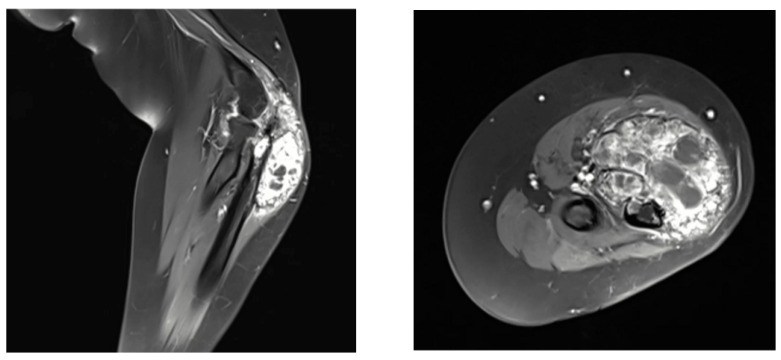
Magnetic resonance imaging (MRI) of the right forearm showing the giant cell-rich osteosarcoma (GCRO).
